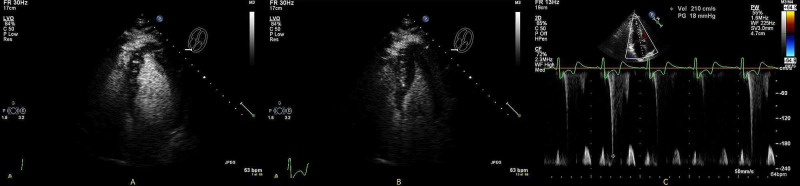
Transthoracic echocardiogram showing apical hypertrophy Apical 4-chamber view in end-diastole (A) and at end-systole (B) showing characteristic “ace of spades” appearance with narrowing of left ventricular apex. (C) Pulsed wave doppler tracing at left ventricular apex demonstrating pressure gradient.
